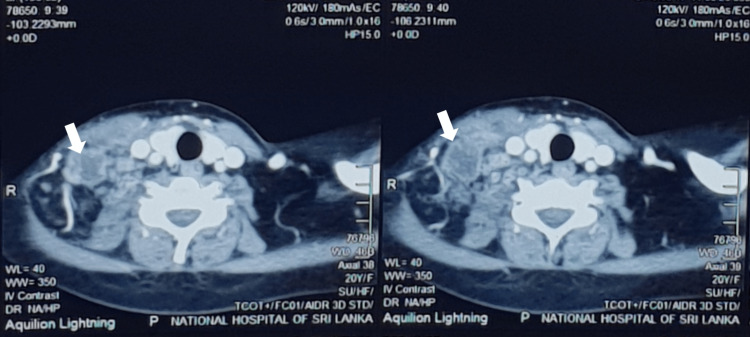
CECT chest and neck CECT: contrast-enhanced computed tomography Arrows indicate right level 4 anterior cervical lymphadenopathy
